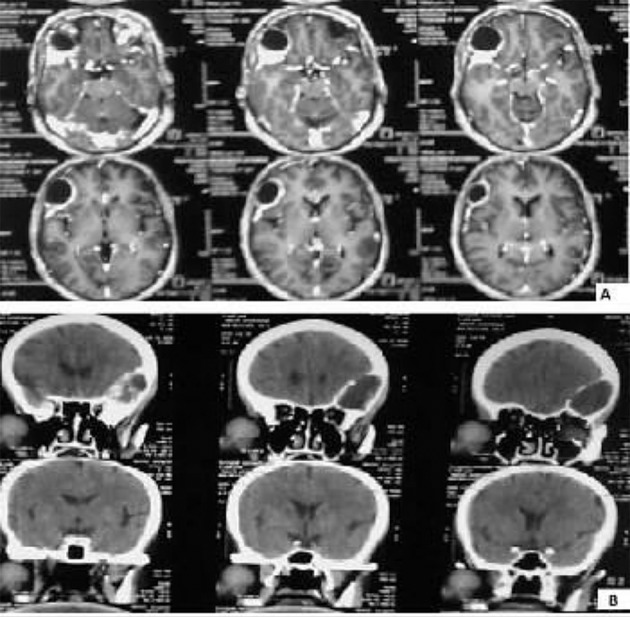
Computed Tomographic Images of the Frontal Bone Cyst Mass
